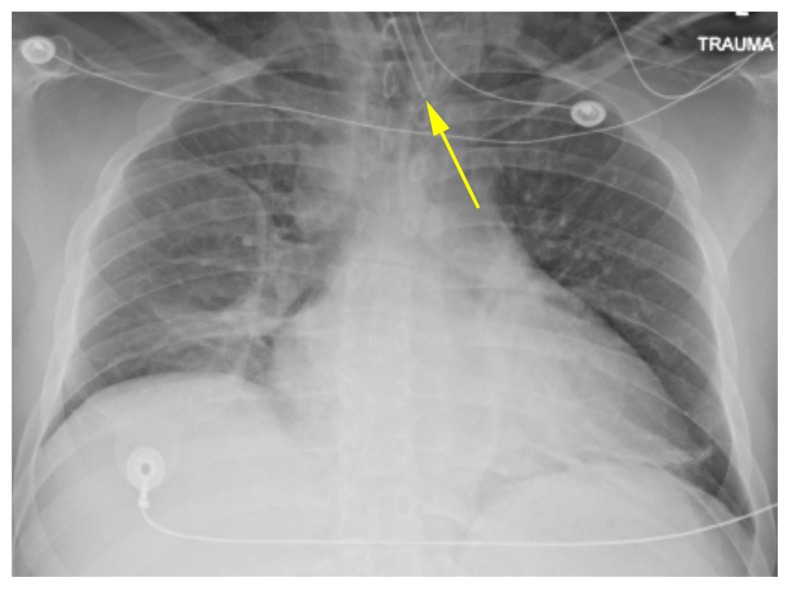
Chest x-ray depicts the ET tube placement.
